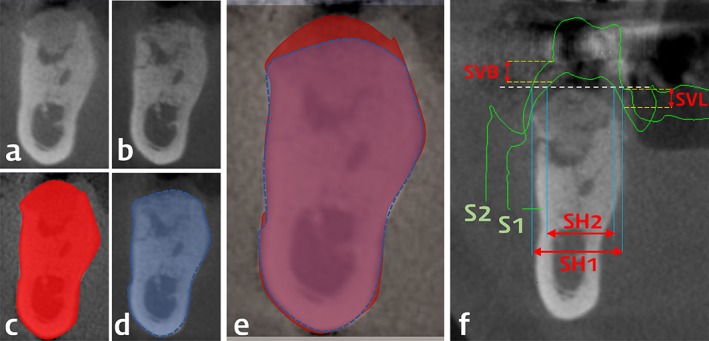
Representative radiographs and overlapped 3D‐scanned study casts (the same case with Figure 1) and linear measurements. Cross‐sectional view from computed tomography taken at the time of surgery (A and C) and 4 months after the surgery (B and D). In the automatically superimposed images from the two time‐points (E), vertical and horizontal changes of the alveolar bone dimension were measured. Surface information from the 3D‐scanned study casts (S1 at the time of surgery; S2 at 4 months after the surgery) were superimposed with the computed tomography at 4 months after the surgery (F). In this view, horizontal soft tissue dimension was measured at the most‐crestal level of the regenerated alveolar bone at two time‐points (SH1 and SH2), and horizontal reduction was calculated by their subtraction. Vertical change of soft tissue dimension was measured at both buccal and lingual gingival margins around the extracted tooth (SVB and SVL)
